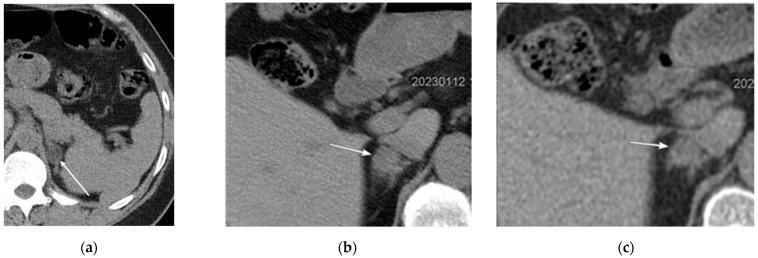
Incidental findings of adrenal nodules on an axial low-dose computed tomography (LDCT) scan. (a) An axial LDCT scan of a 52-year-old female demonstrating a left adrenal adenoma with fat density measuring 17 × 11 mm (arrow). (b,c) Axial LDCT scans of a 46-year-old male demonstrating a stable right adrenal nodule measuring 1.8 cm (arrow) with no interval change observed in the follow-up CT 14 months later (shown in (c)).
